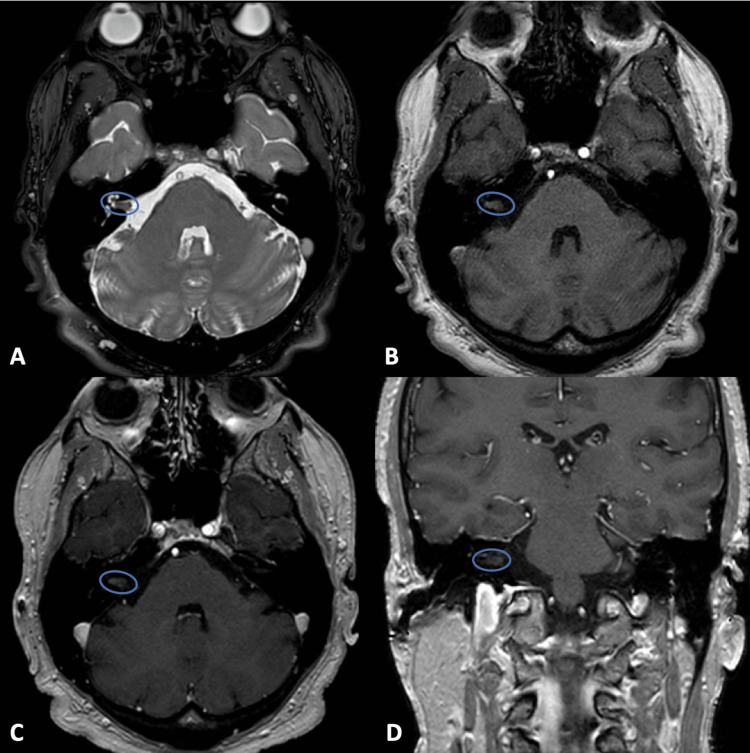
Preoperative magnetic resonance imaging (MRI) A-C: Axial T2-weighted (A) and T1-weighted fast field echo pre-contrast (B) imaging, and axial (C) and coronal (D) T1 post-contrast imaging demonstrating a T2 and T1 isointense lesion (blue circle) without contrast enhancement located anteriorly in the internal auditory canal. No evidence of significant bony remodeling or expansion of the IAC.
